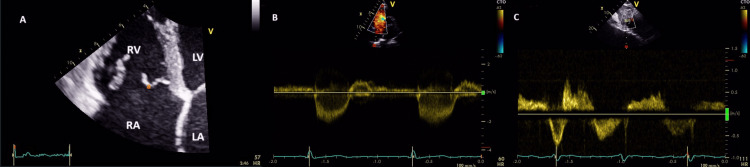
Transthoracic echocardiogram images A: Four-chamber view showing a dilated RV (right ventricle) and prolapse of the tricuspid septal leaflet (a); B: laminar tricuspid flow; C: reversal of the systolic flow in the hepatic veins showing the extent of the tricuspid regurgitation RA: right atrium, LA: left atrium, LV: left ventricle
